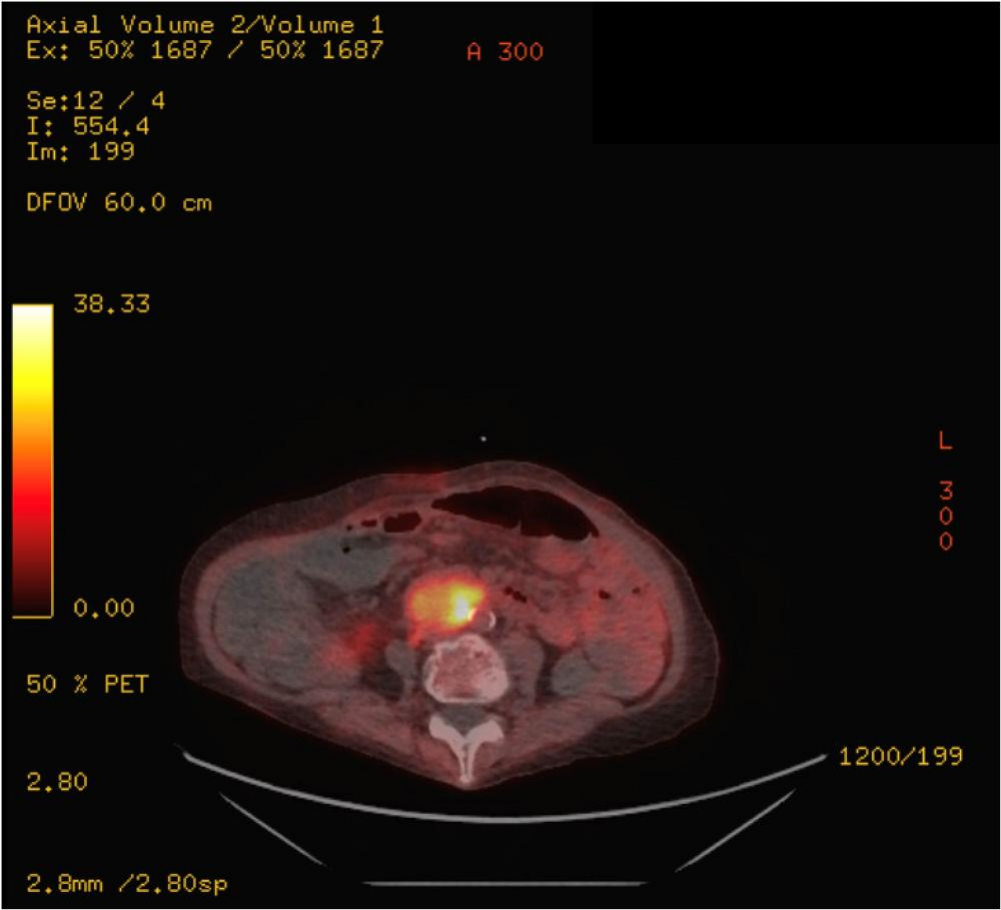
Fluorodeoxyglucose–positron emission tomography (FDG-PET) scan imaging of para-aortic mass. PET imaging showing increased FDG uptake by the para-aortic mass, ultimately found to be a paraganglioma.
